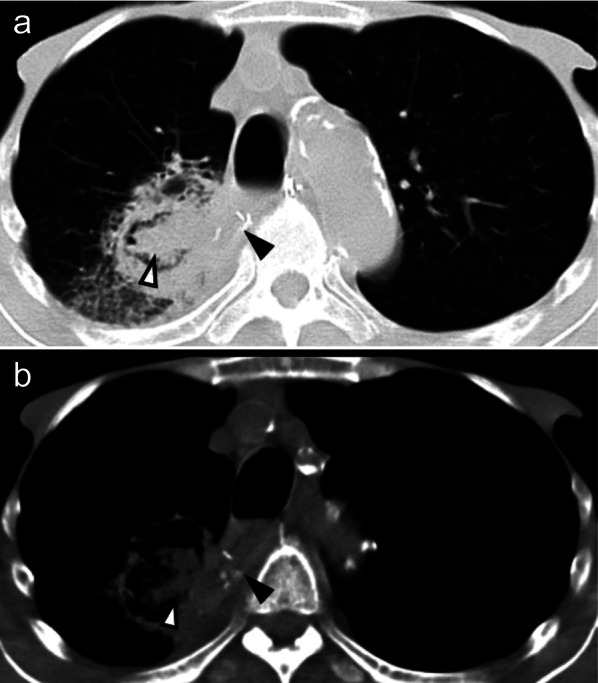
Computed tomography on the 5th postoperative year. a, b anastomotic sites (black arrowhead) and esophagopulmonary fistula (white arrowhead)
